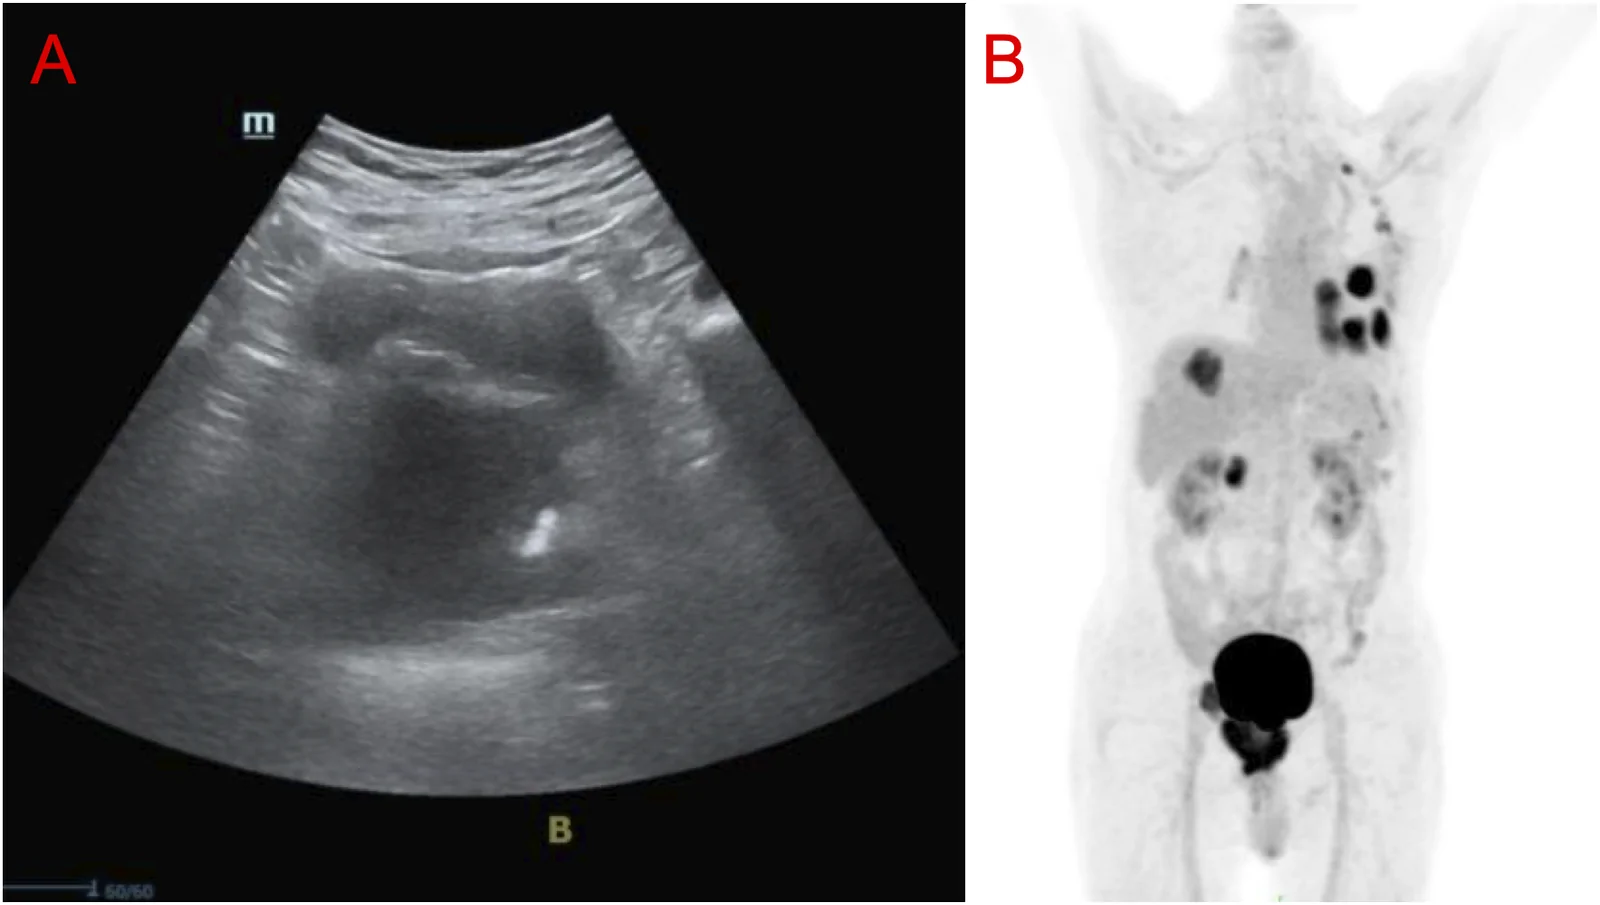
Pelvic ultrasound and whole-body PET-CT showing prostatic enlargement and widespread metastatic disease. (A) Transrectal ultrasound showing a 6.0 × 7.0 × 5.5 cm prostate mass with heterogeneous echotexture. (B) Whole-body PET-CT demonstrating widespread metastatic lesions involving the pleura, seminal vesicle, pelvis, liver, pancreas, and prostate.
